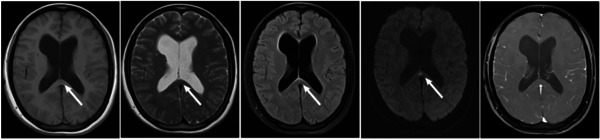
On September 18, brain magnetic resonance imaging image revealed that there were flake long T2 signals in the right basal ganglia, FLAIR showed low signals, small flake long T2 signals in the splenium of corpus callosum, DWI showed high signals (white arrow), and there was no enhancement on enhanced examination.
